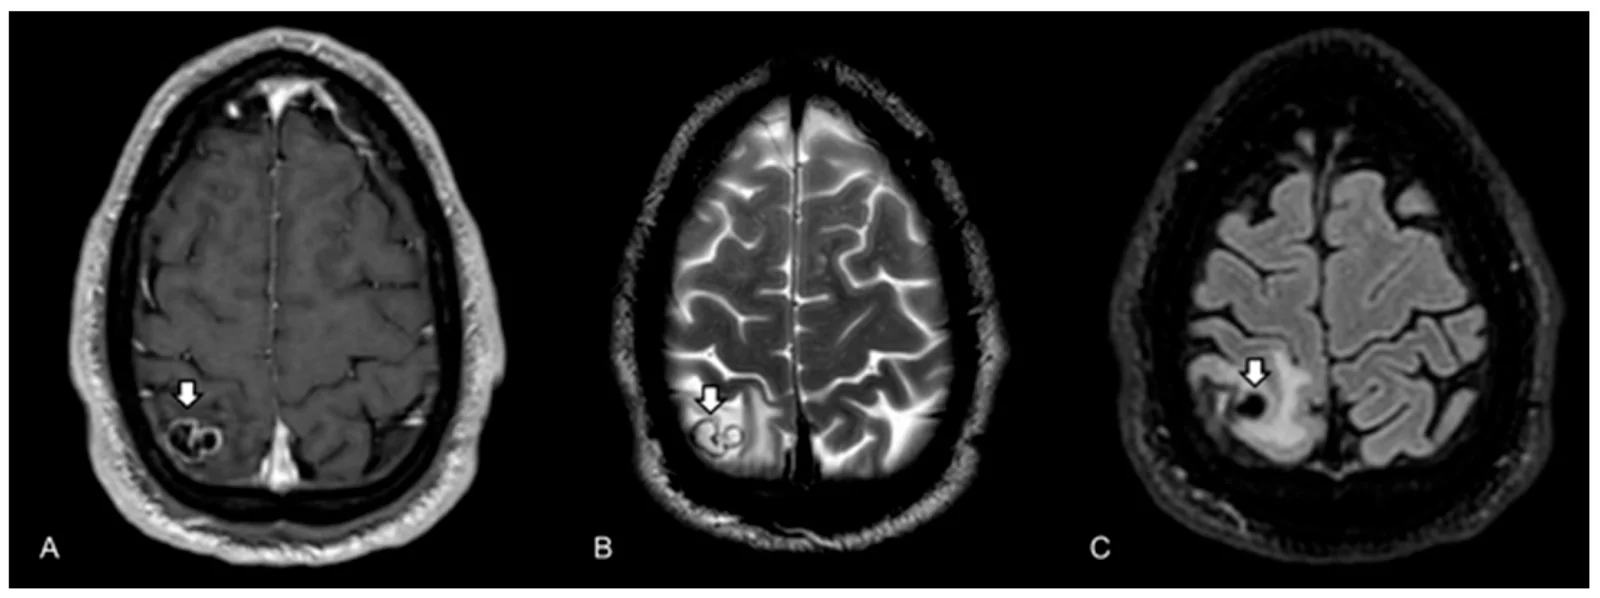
(A) Axial post-contrast T1-weighted image demonstrates a lobulated cystic lesion in the right parieto-occipital region with peripheral ring-like enhancement (arrow). (B) Axial T2-weighted image demonstrates the cystic lesion and surrounding vasogenic oedema, with decreased signal intensity along the lesion wall (arrow). (C) Axial fluid-attenuated inversion recovery (FLAIR) image demonstrates extensive surrounding white matter oedema and decreased signal intensity within the lesion (arrow).
